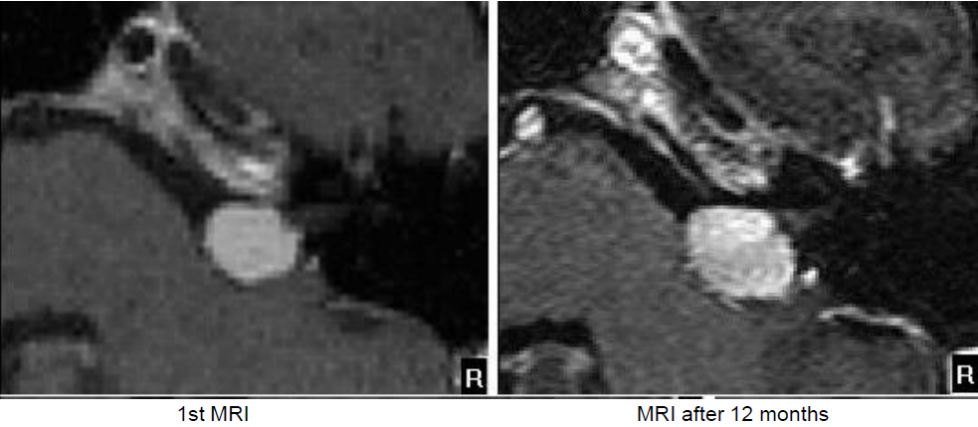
The MRI follow-up examinations (T1-w + CM) show an increased tumor after 1 year.
